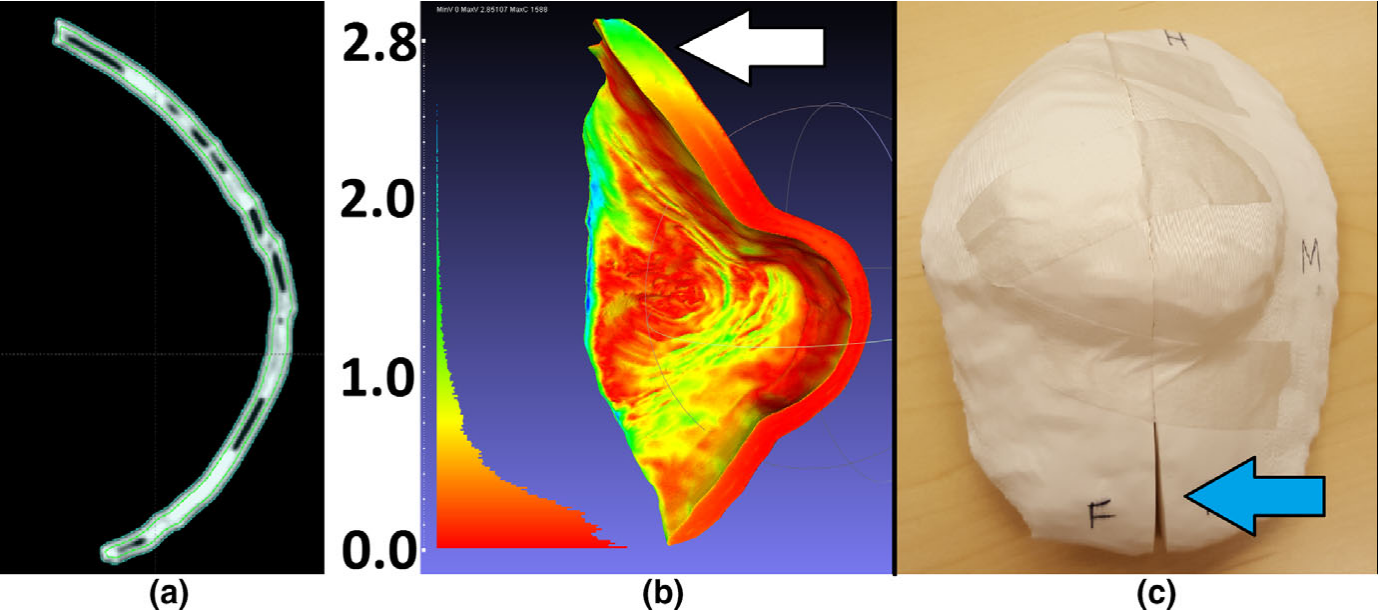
Bolus QA results of interest in the first 10 patients. a) The voids resulting from attempting to print a thin shell with a large nozzle diameter and large extruded width. These voids caused the average CT number to deviate from the expected value. b) A discrepancy due to warping in a flat surface. This resulted in a gap between two bolus pieces that were supposed to fit together, as shown in c). QA, Quality Assurance.
